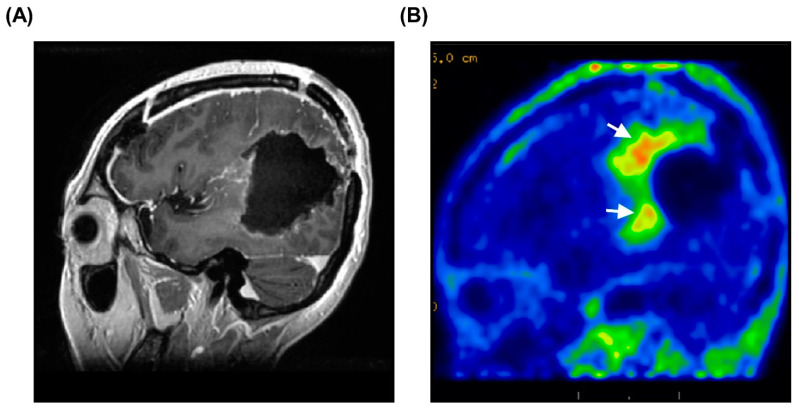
Invisible tumor activity revealed by 18F-BPA-PET. (A) MRI of patient after surgical resection of brain tumors. Postoperative MRI did not identify overt lesions after surgery. (B) Postoperative 18F-BPA-PET revealed strong tumor activity in the surgical margin. The white arrows indicate the infiltrative tumor lesions.
